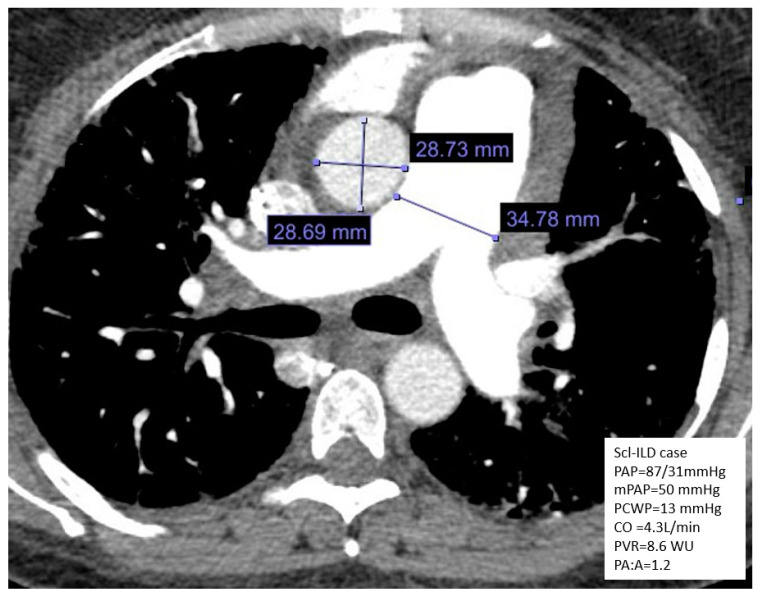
Axial view of CT angiogram of the chest with measurement of the pulmonary artery and aorta in a patient with ILD demonstrating a pulmonary artery to aorta ratio of 1.2. The patient’s hemodynamics based on right heart catheterization are shown in the bottom right square. Abbreviations: mPAP—mean pulmonary artery pressure; PAP—pulmonary artery pressure; PA:A—pulmonary artery to aorta ratio; PVR—pulmonary vascular resistance; Scl-ILD—scleroderma-related interstitial lung disease; PCWP—pulmonary capillary wedge pressure.
